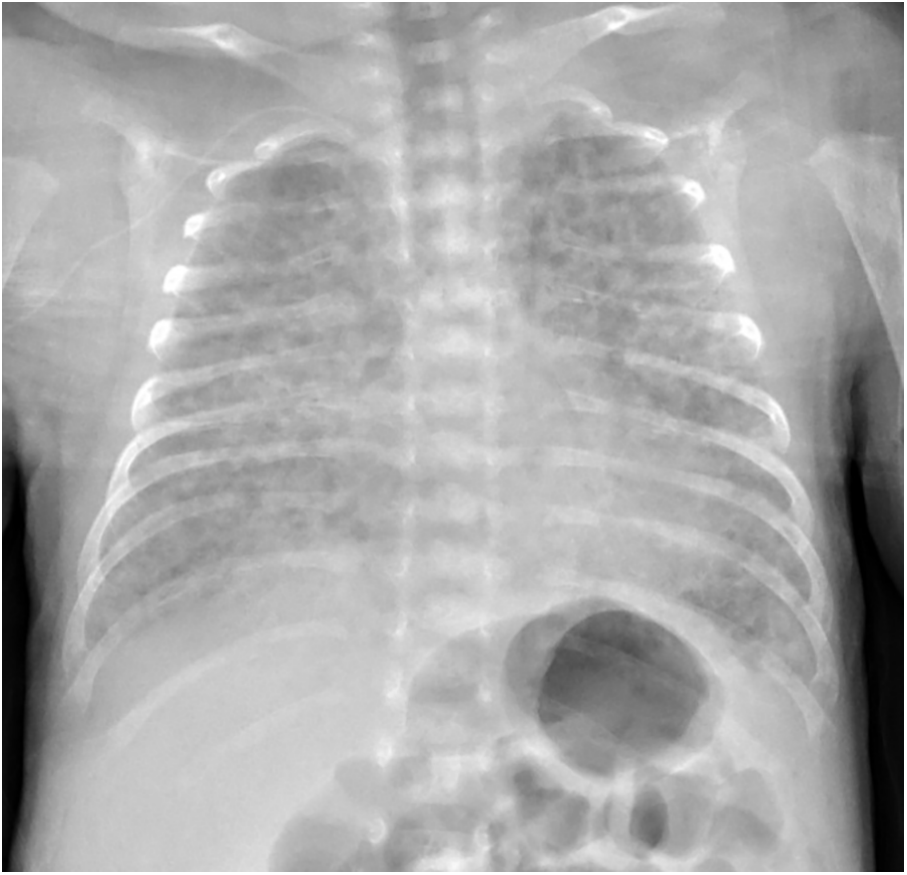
Chest radiograph of an infant with bronchopulmonary dysplasia.
